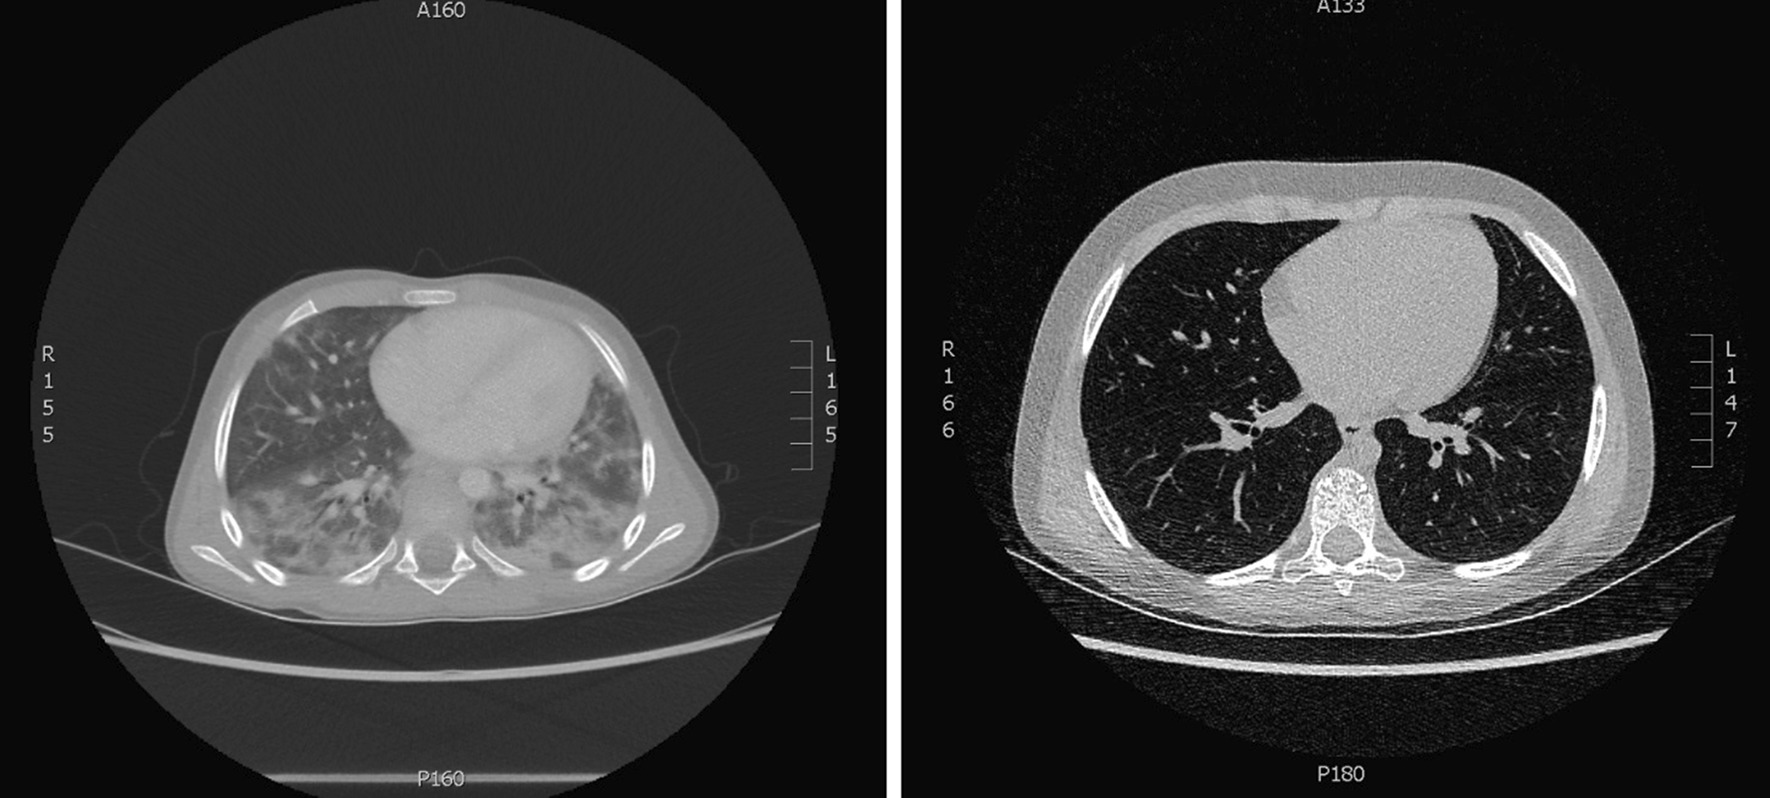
A computed tomography scan of the chest for the time of admission (left) showing bilateral multilobar parenchymal infiltrates, ground glass opacity, interstitial changes, and enlarged hilar lymph nodes however only discrete signs of lung fibrosis with no honeycomb change. After 8 months post high-dose methylprednisolone treatment (right) CT showing complete regression of lung findings
